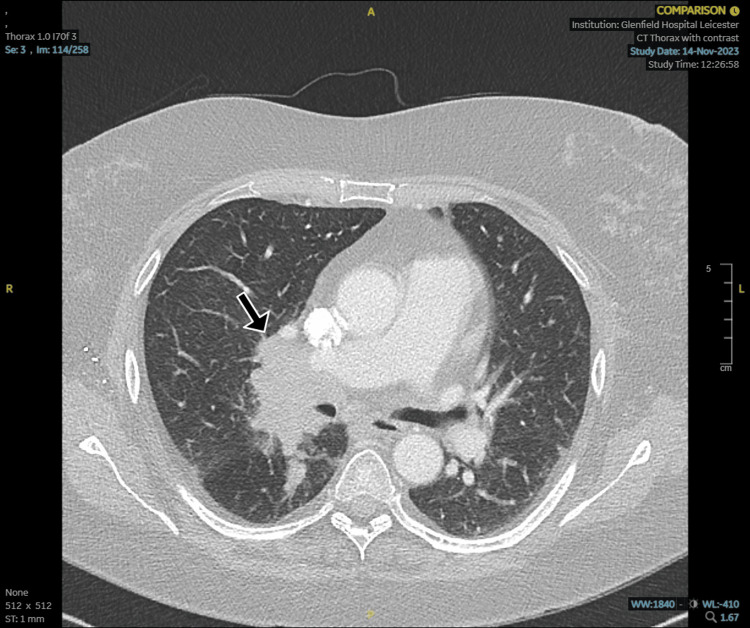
Computed tomography (CT) thorax Contrast-enhanced axial CT of the thorax demonstrating a spiculated right hilar mass (black arrow) measuring approximately 4 cm in greatest diameter. The mass is abutting the right main bronchus and associated with enlargement of adjacent mediastinal lymph nodes. No evidence of pleural effusion, chest wall invasion, or direct mediastinal involvement is seen on this image.
